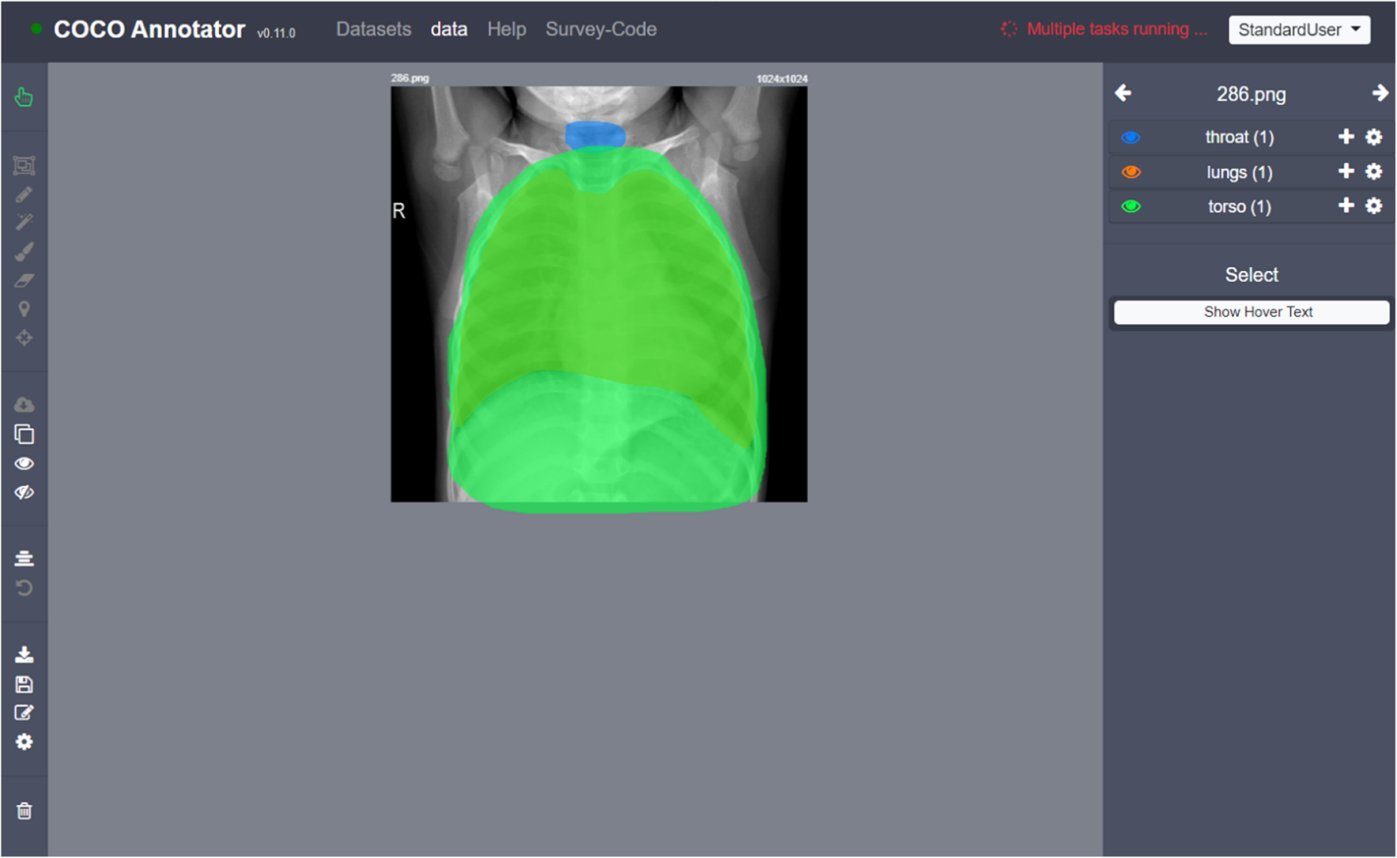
The user interface of the customized COCO Annotator used by the crowdworkers to annotate the images. Using the tools on the left on each X-ray, the areas for the throat, lungs, and torso were highlighted (color figure online)
